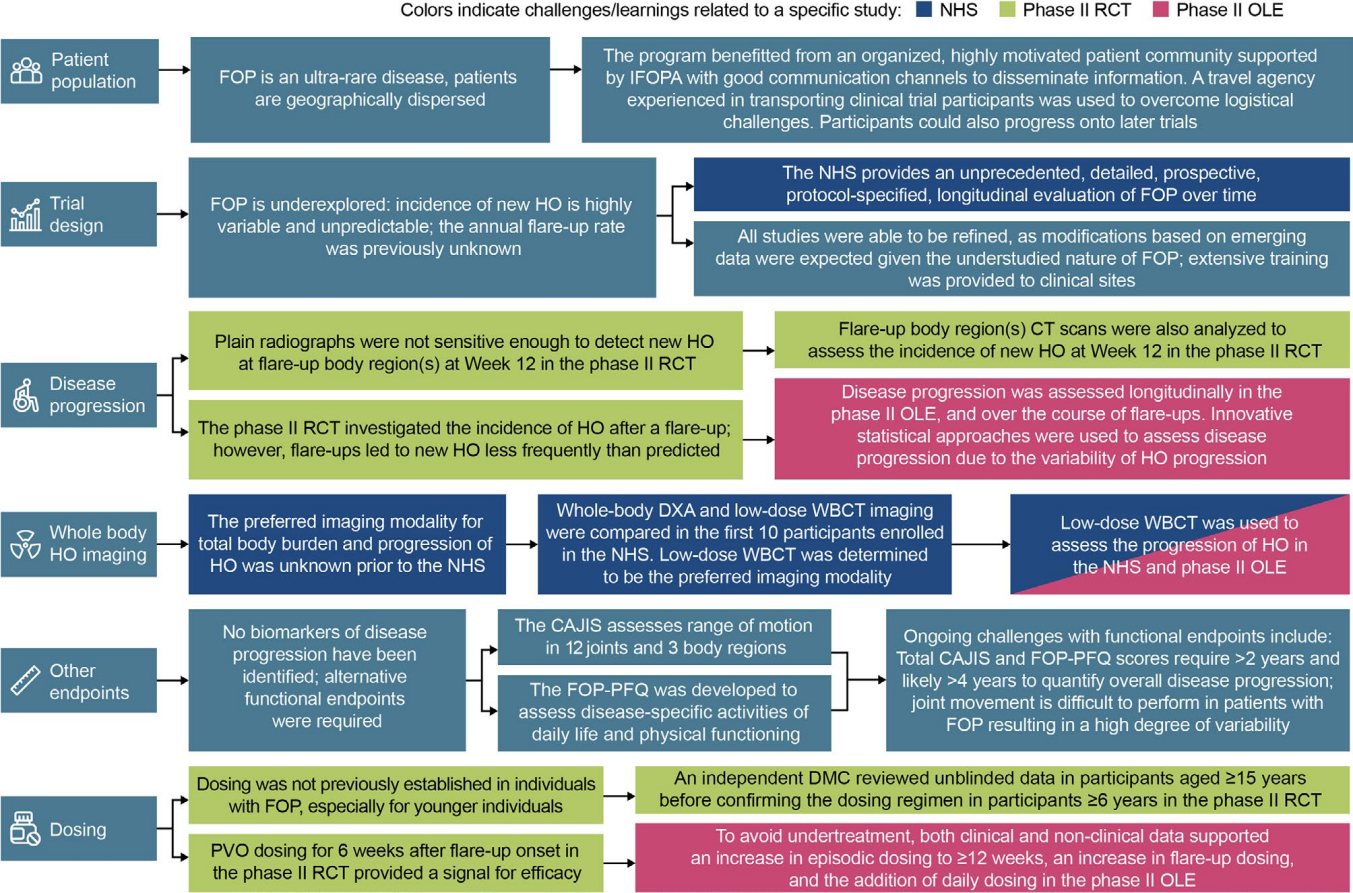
Key challenges and protocol amendments during the PVO-1A-001, PVO-1A-201, and PVO-1A-202 studies. CAJIS: Cumulative Analogue Joint Involvement Scale; CT: computed tomography; DMC: data monitoring committee; DXA: dual-energy X-ray absorptiometry; FOP: fibrodysplasia ossificans progressiva; FOP-PFQ: FOP Physical Function Questionnaire; HO: heterotopic ossification; IFOPA: International FOP Association; NHS: natural history study; OLE: open-label extension; PVO: palovarotene; RCT: randomized controlled trial; ROM: range of motion; WBCT: whole-body computed tomography
